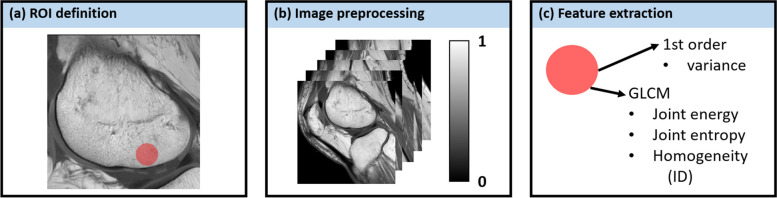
Inter-sequence comparison of bone texture. On the T1-weighted images, radiomic features were analyzed by defining a standardized circular region of interest adjacent to the subchondral lamella underneath the 5-mm defect (a). Image preprocessing included normalization of the signal intensity to the range of 0 to 1 (b). As texture features, variance as a first-order feature and joint energy, joint entropy, and homogeneity (*i.e.*, ID) as gray-level co-occurrence matrix (GLCM) features were extracted (c). For the computation of the GLCM, the normalized images were quantized into 200 evenly spaced bins. *GLCM* Gray-level co-occurrence matrix, *ID* Inverse difference
